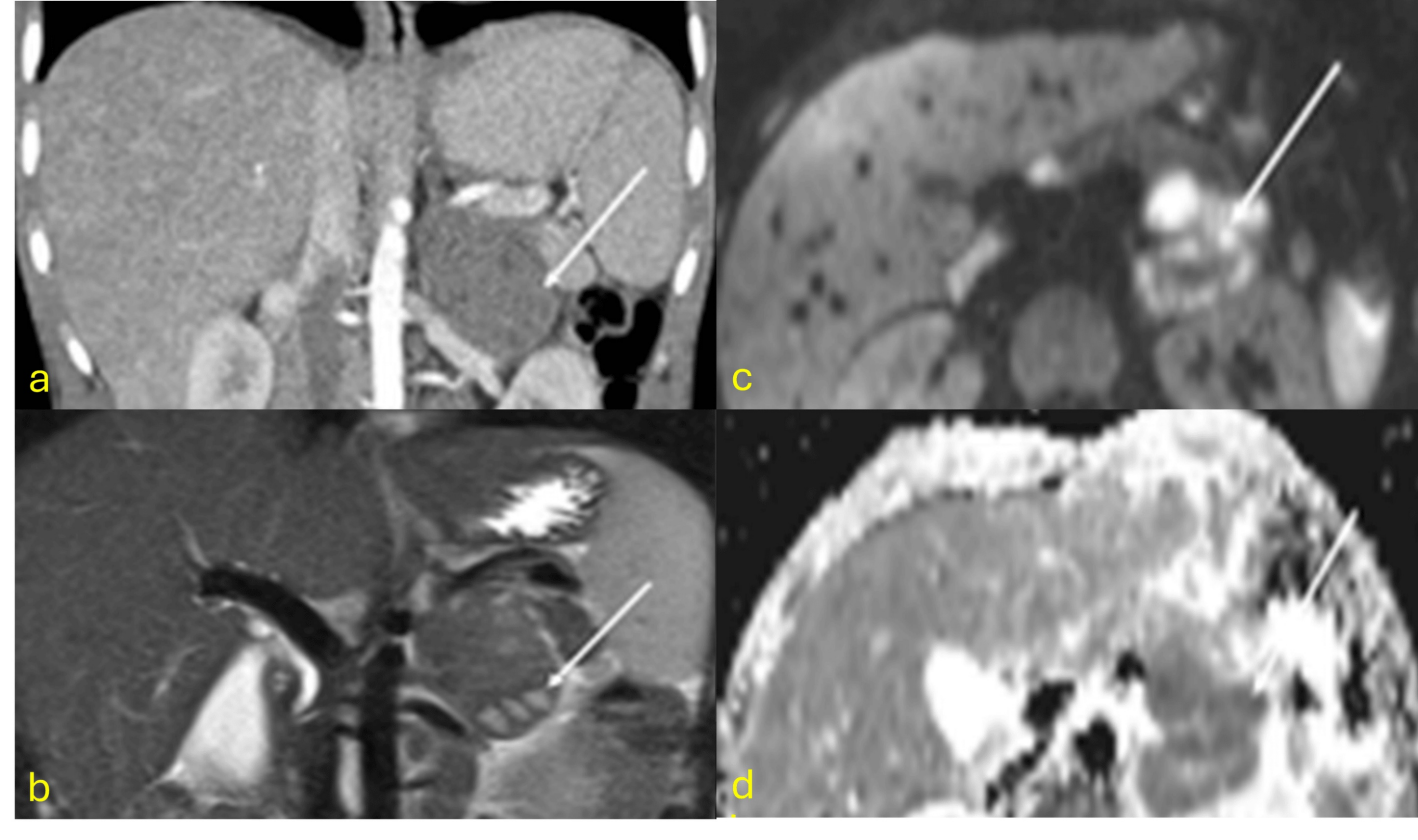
Tuberculous abscess in the left adrenal gland CT: computed tomography; MRI: magnetic resonance imaging (a) Coronal contrast-enhanced CT scan showing a 5 cm hypodense mass in the left adrenal gland with no post-contrast enhancement, in a patient with active pulmonary tuberculosis. (b) T2-weighted MRI and (c and d) diffusion-weighted MRI showing areas of high signal intensity on T2-weighted images and marked diffusion restriction, indicative of crypts (arrows). Diagnosis confirmed by CT-guided biopsy revealing necrotizing granulomatous inflammation and positive GeneXpert MTB/RIF assay
